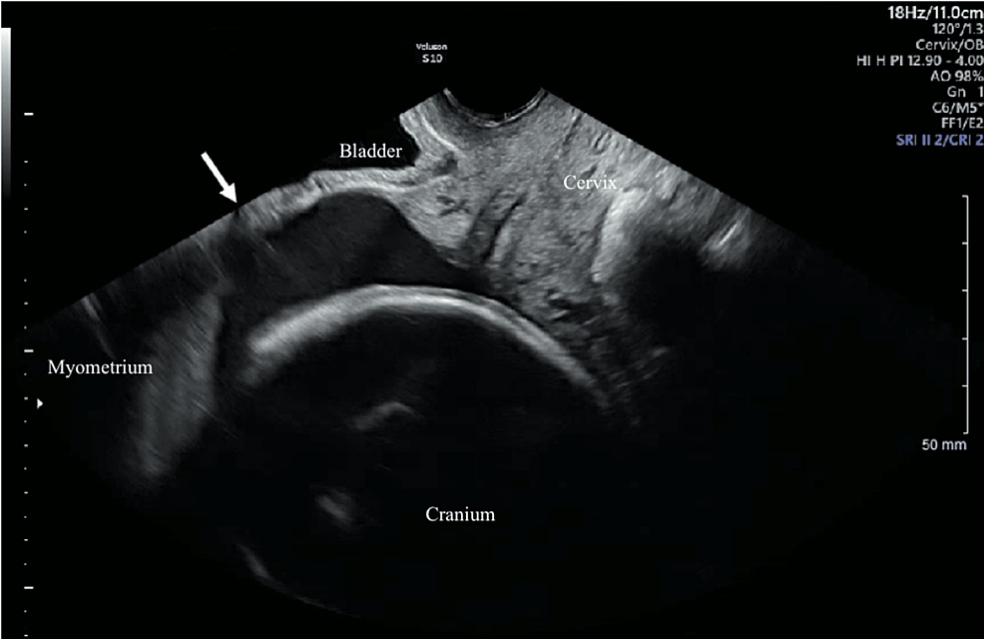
Transvaginal ultrasound of the lower uterine segment. Transvaginal sonographic image of the lower uterine segment re-demonstrating the presence of uterine dehiscence of 3.7 cm in the sagittal view
